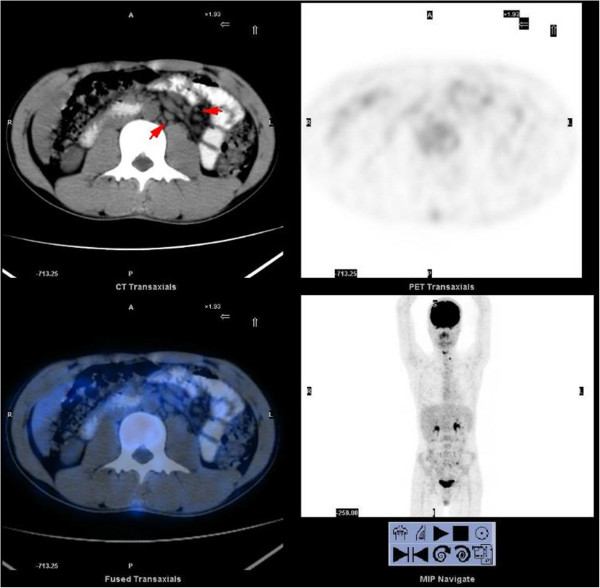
PET-CT revealed multiple enlarged lymph nodes (arrow) in the abdominal and mesenteric region without abnormal concentration of 18 F-fluorodeoxyglucose (18 F-FDG).
